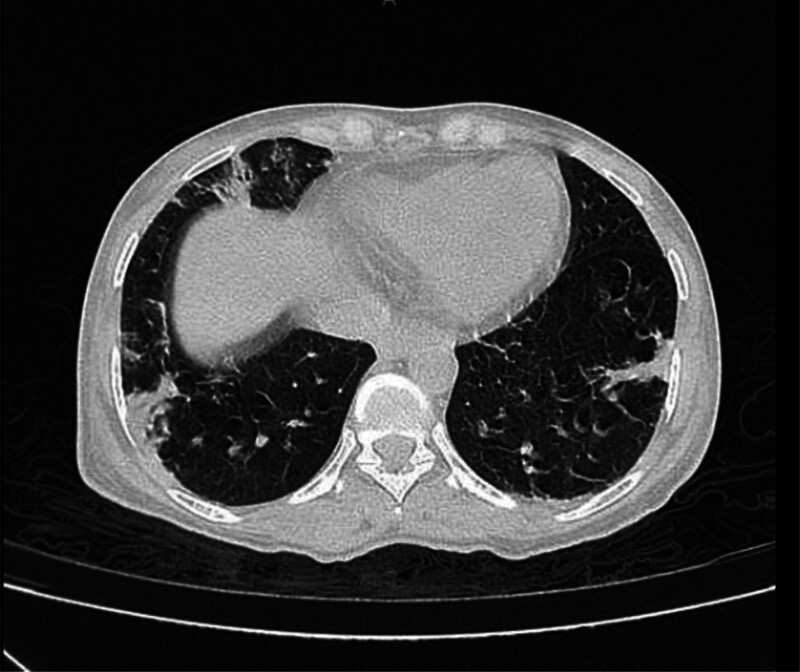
Slice-cut of a pre-contrast CT image of the lungs demonstrating cryptogenic complicated organized pneumonia. CT = computed tomography.
